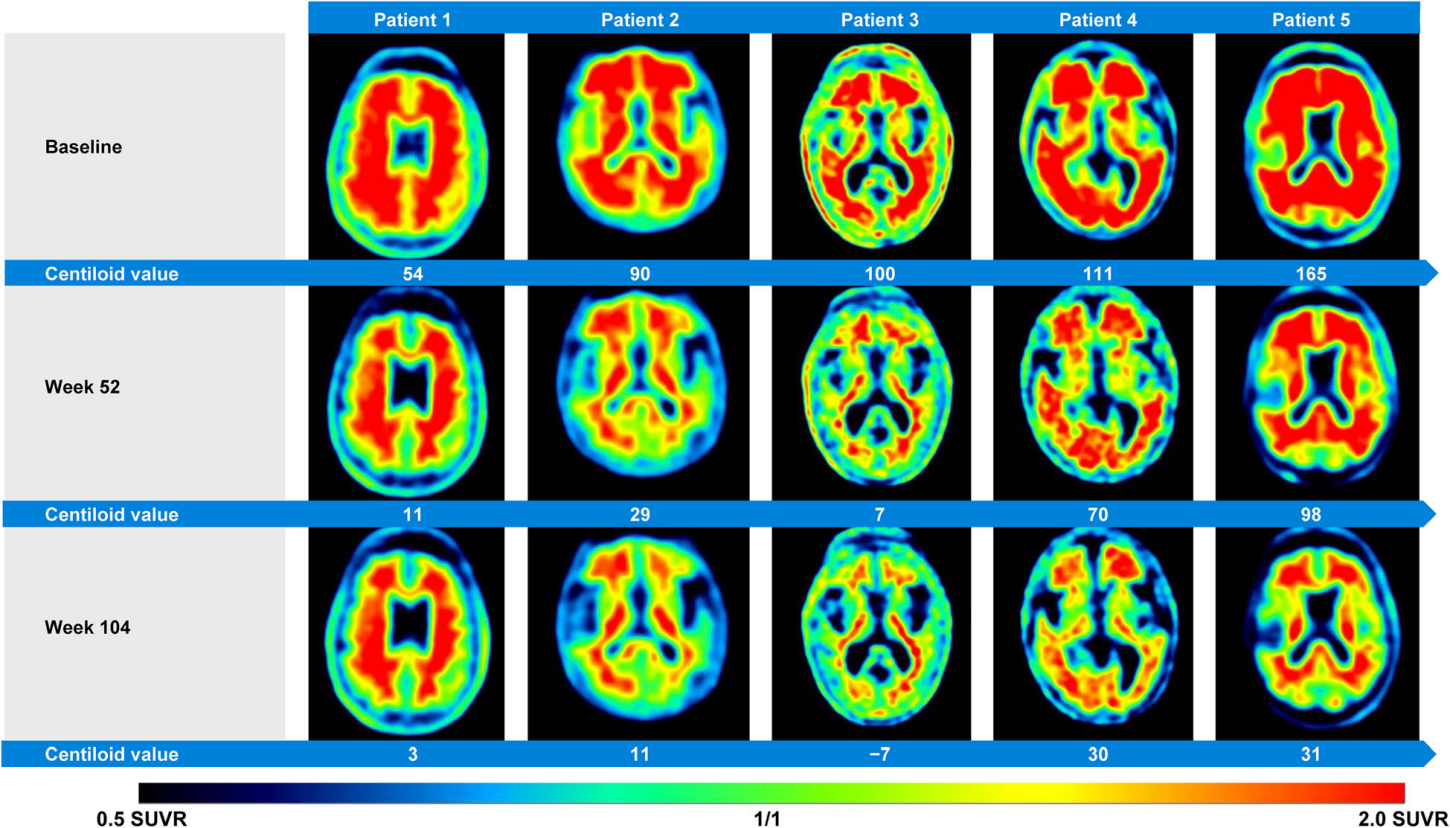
Amyloid-β plaque reduction with gantenerumab. Axial florbetapir brain PET images from five patients displaying reduction of amyloid-β plaques from OLE baseline to OLE week 52 and OLE week 104. Axial slices are at the level of the basal ganglia. PET images were obtained 50 min post-injection, SUVR data with the cerebellar cortex as the reference region
